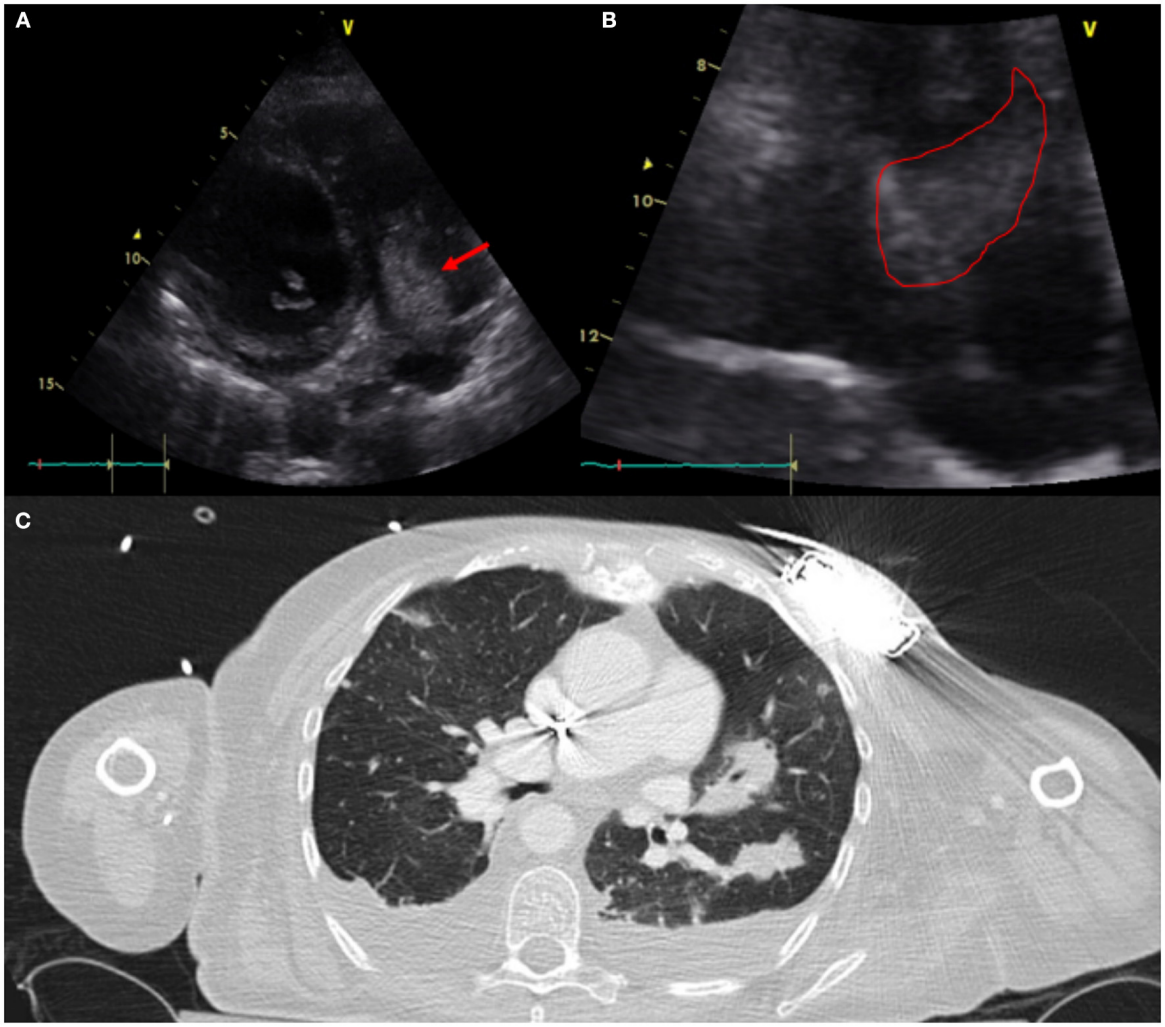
Sixty one year-old woman with a history of NICM s/p single lead ICD who presented with fever and malaise. Infectious work up was positive for MSSA bacteremia. TTE showed a very large vegetation attached to the ICD lead in the RA protruding into the RV [(A)-modified apical view and (B)-RV focused view with RA zoom]. A pre-procedural CT (C) was obtained and revealed bilateral, extensive and multifocal septic emboli with cavitary lesions as well as bilateral pleural effusions without evidence of pocket infection. The patient underwent open sternotomy with device extraction and TV repair for septal leaflet perforation for management of CIED systemic infection with IE.
